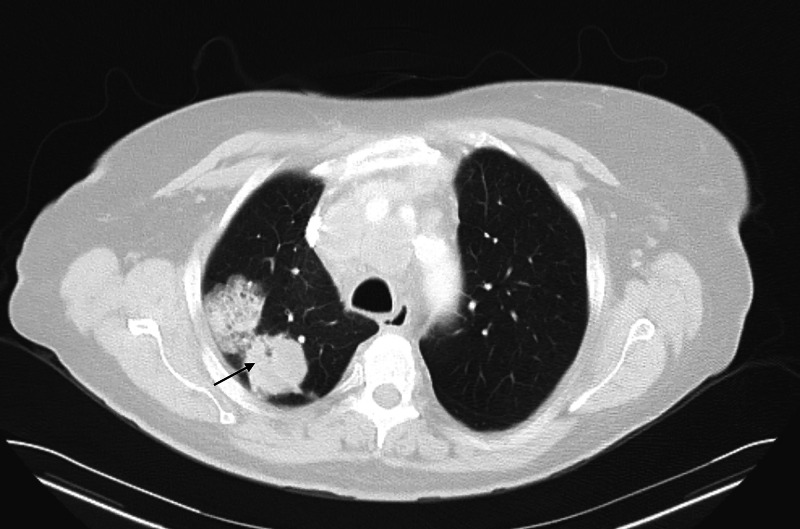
Axial CT scan chest The arrow shows bilobed lung mass in the right upper lobe. CT: computed tomography
